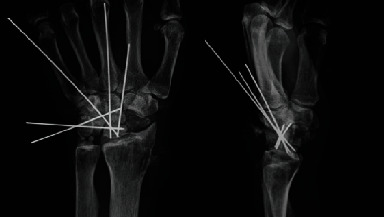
X-ray image obtained immediately after the surgery using the Watson method.
